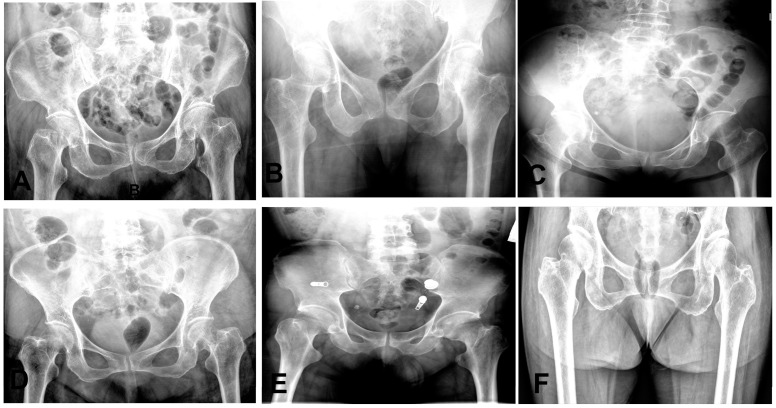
Representative radiographs of six nondisplaced femoral neck fractures initially diagnosed as normal on anteroposterior hip X-rays. (A,F): Right hip fractures (two cases). (B–E): Left hip fractures (four cases).
